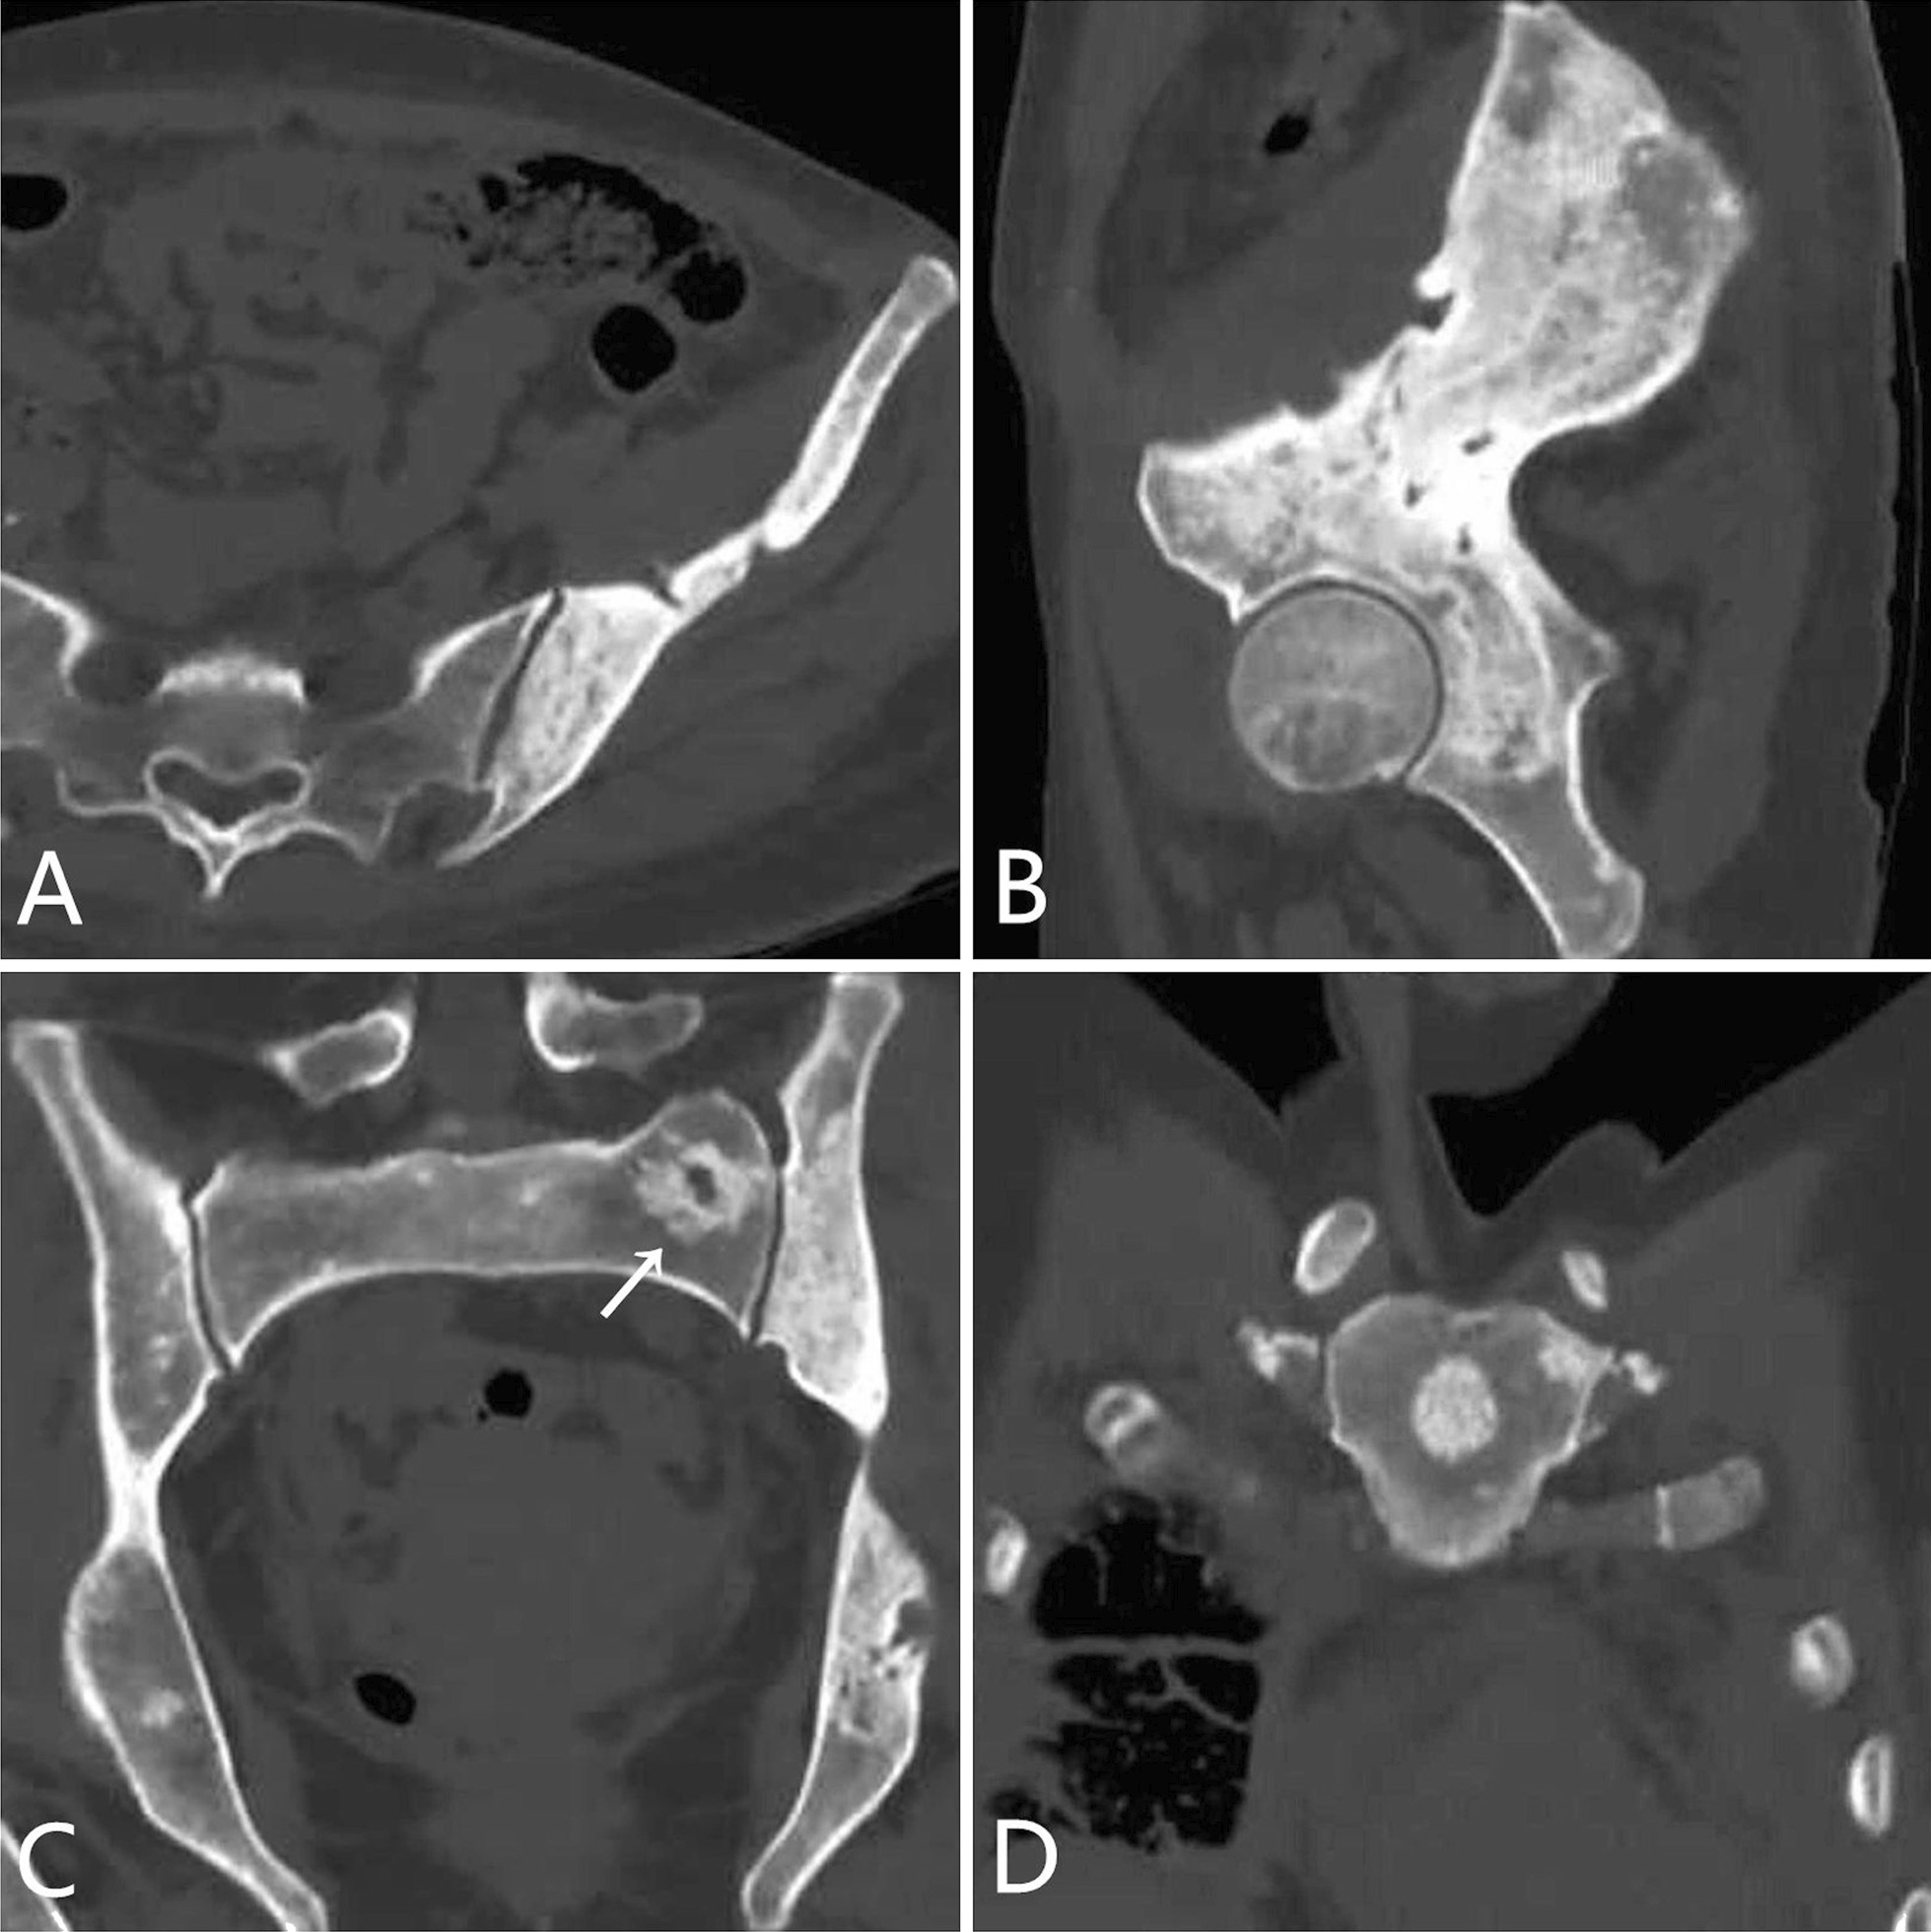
CT images in a 40-year-old woman with pelvis, spine, and sternum involved. A Axial and B sagittal reconstruction pelvic CT images show heterogeneous diffuse osteosclerosis filling the left iliac bone with multiple lucent foci and disseminated and multiple cortical osteolytic lesions. C Coronal reconstruction pelvic CT image shows a lytic lesion with sclerotic margin in the sacrum, looking like a ring (arrow). D Coronal reconstruction chest CT image shows solitary well-circumscribed, nodular osteosclerotic lesion in the sternum
